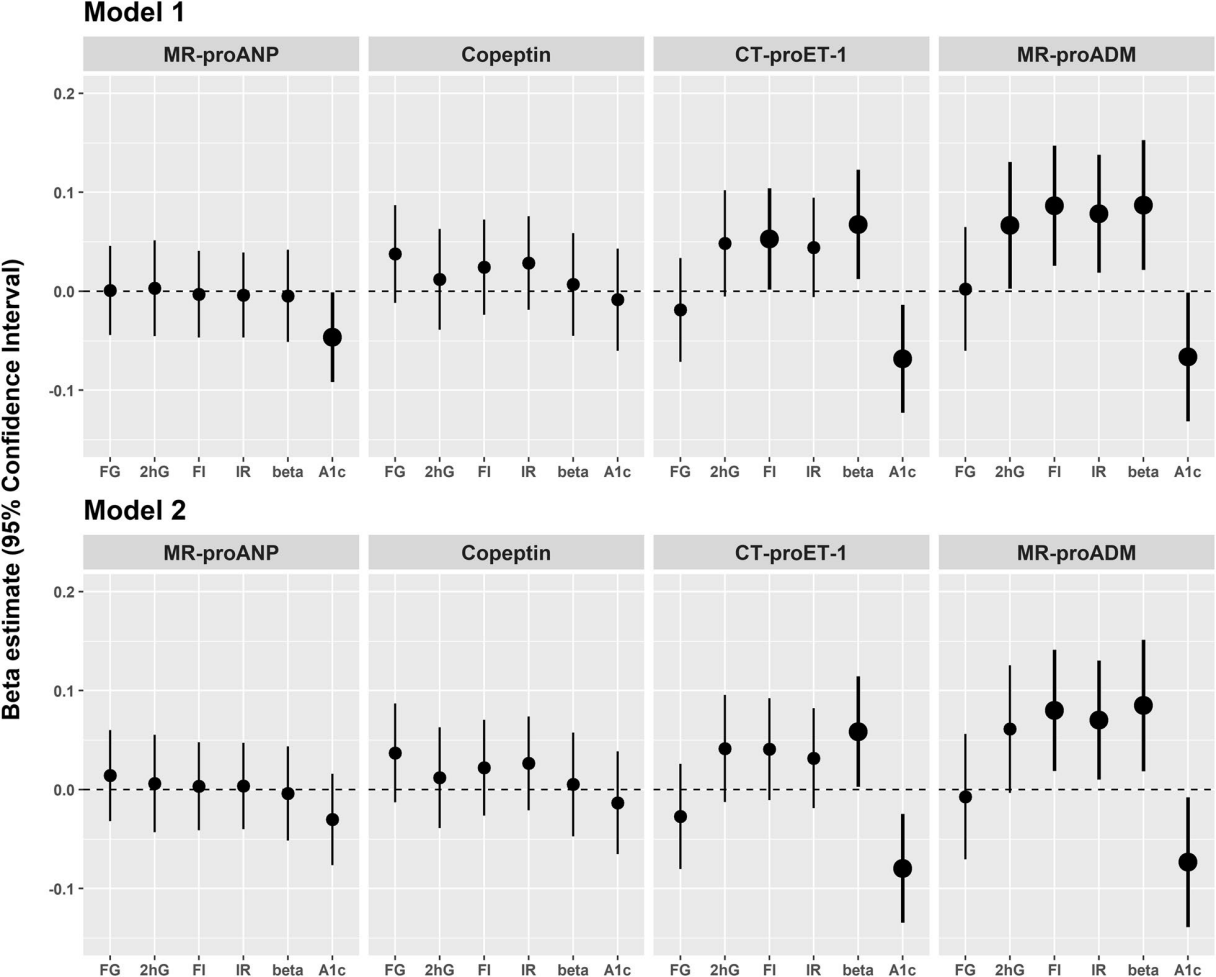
Associations of cardiac stress biomarkers with continuous traits of glycaemia and insulin resistance at follow-up. Thick plots show significant associations. The analyses were adjusted for baseline value of glycaemic or insulin traits, age, sex, waist circumference and height (model 1) and were further adjusted for actual hypertension, ratio of total cholesterol and HDL, triglycerides, smoking status, physical activity and parental history of diabetes (model 2). Abbreviations: 2 hG, 2 h-glucose; A1c, haemoglobin A1c; beta, homeostasis model assessment of beta-cell function; CT-proET-1, C-terminal pro-endothelin-1; FG, fasting glucose; FI, fasting insulin; IR, homeostasis model assessment of insulin resistance; MR-proADM, mid-regional pro-adrenomedullin; MR-proANP, mid-regional pro-atrial natriuretic peptide
